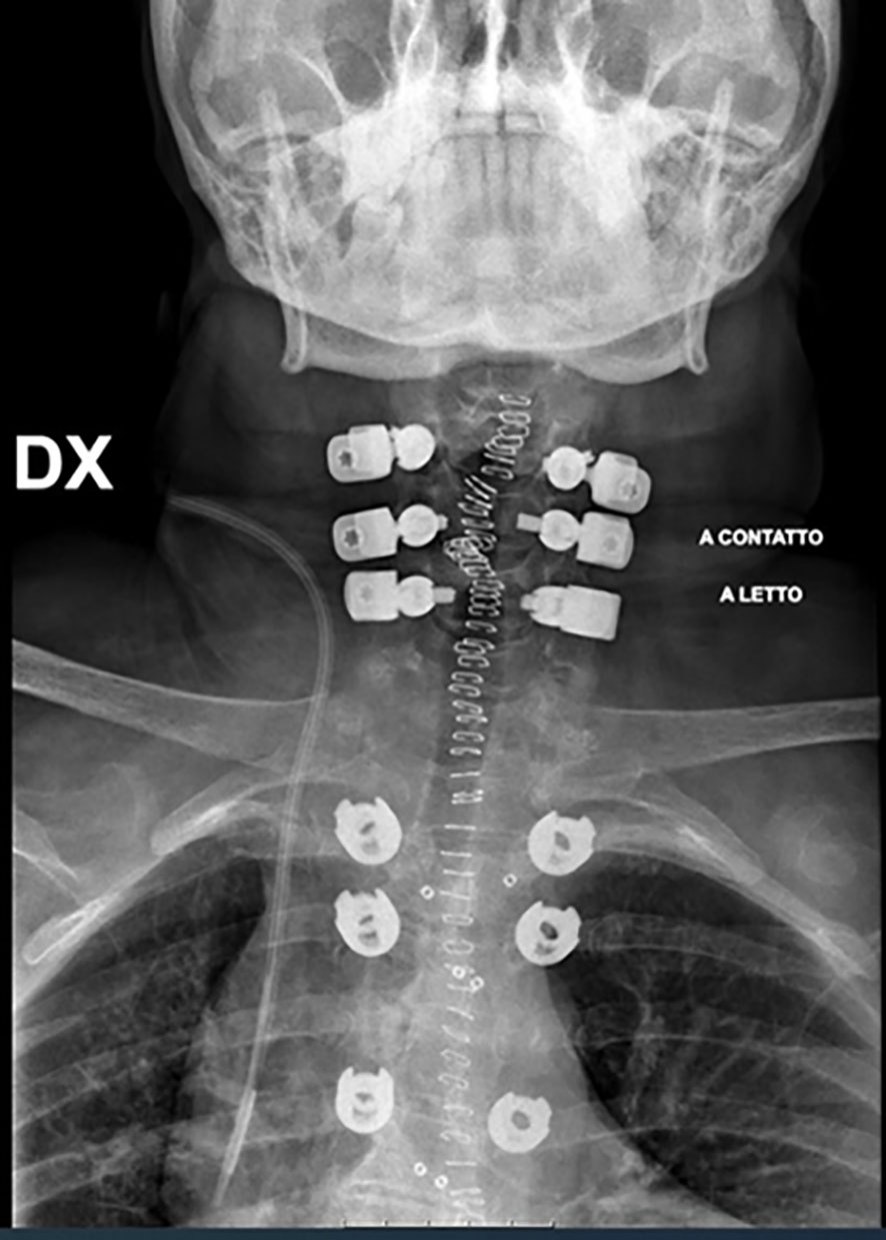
Radiograph of final construct.
